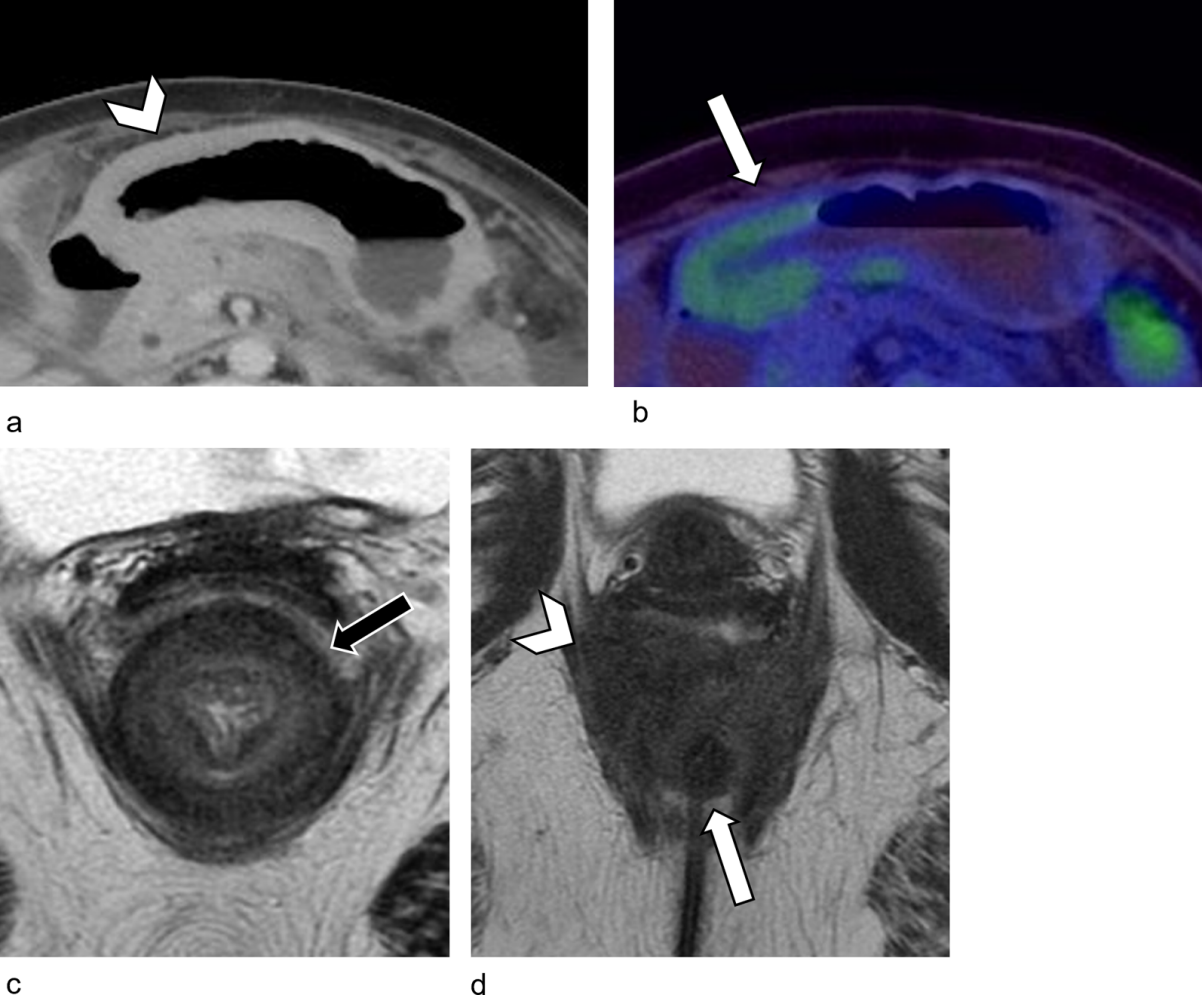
Linitis plastica appearance of the stomach and rectum due to metastatic ILC in a 61-year-old female. Six years after completing therapy for Stage 1A ILC of the right breast, she presented with change in bowel habit and a palpable rectal mass on examination. She later developed symptoms of subacute bowel obstruction. a Axial contrast-enhanced CT image shows incidental diffuse, circumferential wall thickening of the stomach (white arrowhead), suggestive of linitis plastica. b Corresponding axial PET/CT demonstrates mild ^18^F-FDG-uptake (SUVmax 3.05) in the distal stomach (white arrow). This degree of metabolic uptake was deemed at the upper limit of physiological uptake. Diffuse metastatic involvement of the stomach was confirmed on exploratory laparotomy. c Axial T2-weighted MR image demonstrates circumferential thickening of the rectum, with preserved concentric bowel wall layers (black arrow), giving a “target sign” appearance. d Axial T2-weighted MR image of the pelvic floor shows a near-circumferential, ill-defined, T2-weighted hypointense mass (white arrowhead) around the anal canal (white arrow). Initial biopsies were inconclusive, likely due to the predominantly extramural location of the mass. Metastatic involvement was confirmed on subsequent deep biopsy
